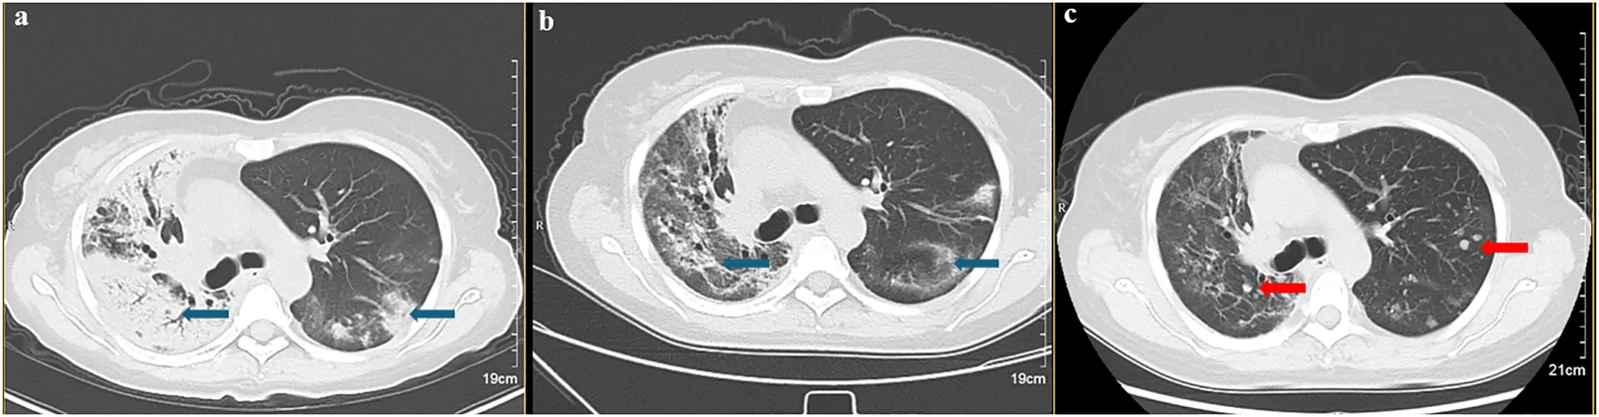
Chest CT scan showing cord-like interstitial thickening in the middle lobe (arrow), indicating interstitial lung disease (ILD) during the 5^th^-line treatment with T-DXd and pembrolizumab (a). ILD was (b) partially resolved (arrow) 2 months after discontinuation of T-DXd, treatment with meropenem, methylprednisolone and nintedanib, and (c) ILD was completely resolved (arrow) 5 months after ILD treatment.
